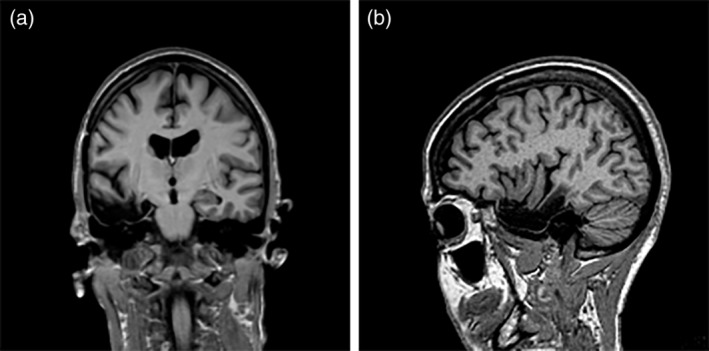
MRI scans, taken 15 months after surgery, show the resection size (i.e., 6 cm from the anterior temporal pole in posterior direction, 5 cm on the left–right axis, and 2 cm on the dorsoventral axis). The hippocampus head, body, and part of the tail were resected. The anterior part of the amygdala was spared. All but the anterior and medial rim of the amygdala turned into gliotic tissue, possibly due to ischemia. Furthermore, the anterior part of parahippocampal gyrus (leaving a small medial rim), the anterior part of the fusiform gyrus, the anterior inferior part of the superior temporal gyrus, the anterior part of the middle temporal gyrus, and the inferior temporal gyrus were also resected. This includes the entorhinal and transentorhinal region. (a) Coronal view, (b) sagittal view. Later scans (4 and 12 years after surgery) showed that the lesion area has not changed over time; nor were there any indications of atrophy or other brain pathology. The right side of the brain in the coronal image corresponds with the left side
